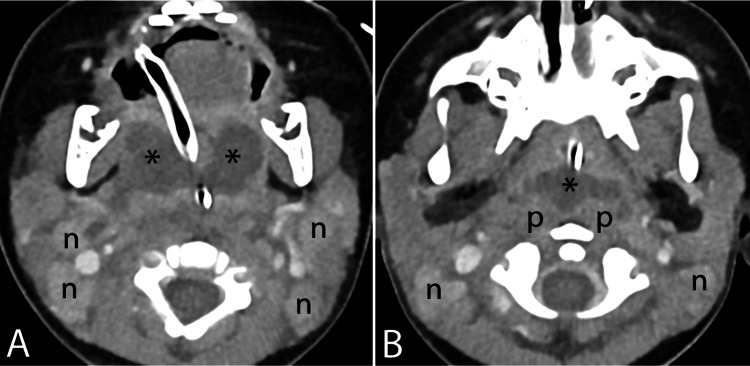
First CECT of the child showing axial planes at the level of the palatine tonsils (A) and slightly more superiorly at the pterygoid plates (B), with endotracheal and nasogastric catheters in place. Bilateral paired collections with rim enhancement were observed in the tonsillar fossae (asterisks in A), positioned directly lateral to the endotracheal catheter, replacing the normal soft tissue density of the palatine tonsils. The anterior extent of the collection reached the base of the tongue, while inferiorly it extended to the valleculae, just superior to the hyoid bone. Superiorly, adenoid hypertrophy was noted (not shown). Posterolaterally, fluid hypodensity was observed extending into the carotid spaces without rim enhancement. Posteriorly, part of the collection extended into the retropharyngeal space (asterisk in B), adjacent to the prevertebral longus coli muscles (marked as p), which appeared edematous but not infiltrated. There was no extension of the retropharyngeal collection inferiorly into the oropharynx or superior mediastinum. The palatine and lingual tonsils were not well delineated. Multiple enlarged enhancing cervical lymph nodes were present bilaterally at levels IIa and IIb (marked as n). CECT, contrast-enhanced CT
